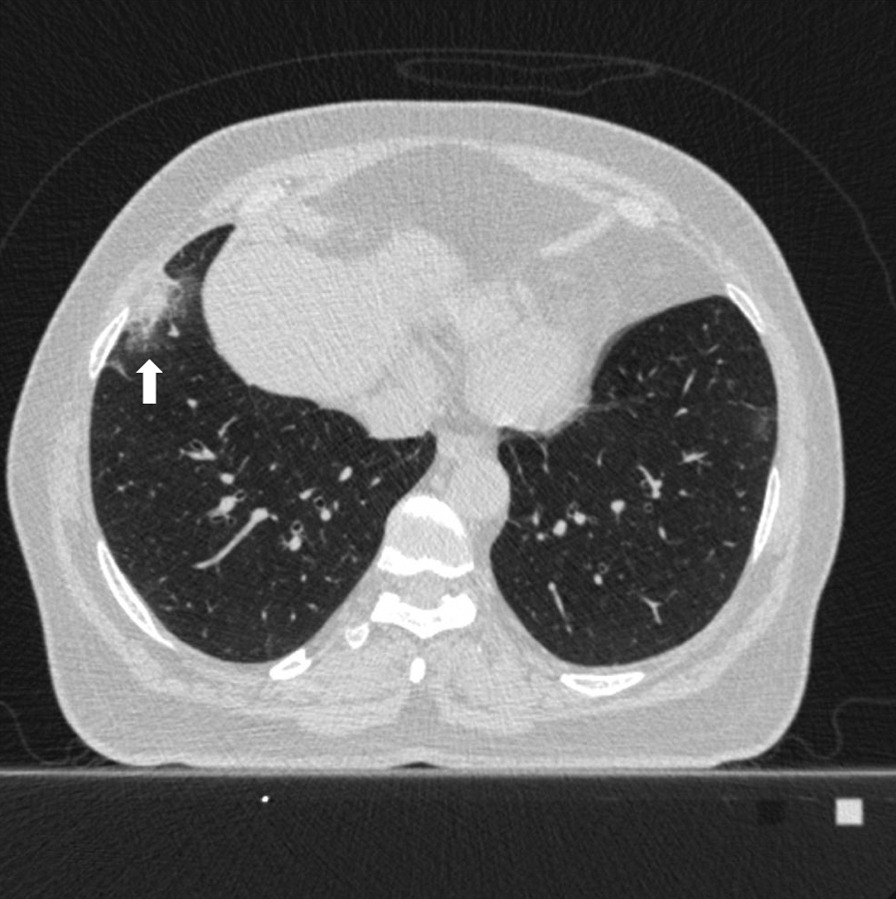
48 years old female patient. According to the CO-RADS category in terms of Covid pneumonia, a CO-RADS 4 patient had a mixed parenchyma pattern (pattern 2) with a peripheral lesion (white arrow), and the patient's total CT score value was 2 and the structured total CT score value was 4 according to HRCT scan
